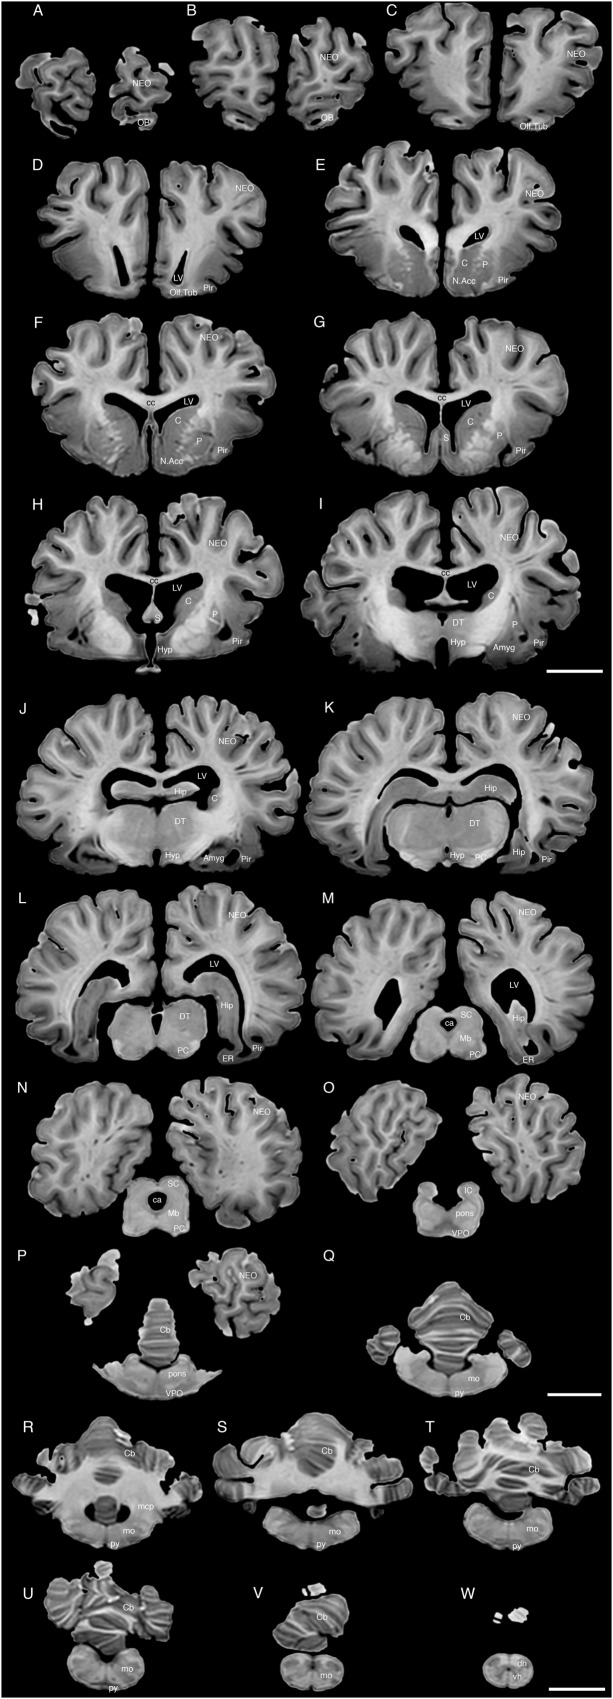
A series of coronal structural MR images through the brain of the white rhinoceros (*C. simum*). (A) is the most rostral section and (W) is the most caudal section, with each section having a thickness of 2 mm and each section being 6 mm apart. Note the typically mammalian topography of the various regions and structures of the brain. Scale bar = 2 cm. Amyg, amygdaloid body; C, caudate nucleus; ca, cerebral aqueduct; Cb, cerebellum; cc, corpus callosum; dh, dorsal horn; DT, dorsal thalamus; EC, entorhinal cortex; Hip, hippocampus; Hyp, hypothalamus; IC, inferior colliculus; LV, lateral ventricle; N.Acc, nucleus accumbens; NEO, neocortex; Mb, midbrain; mcp, middle cerebellar peduncle; mo, medulla oblongata; OB, olfactory bulb; Olf. Tub, olfactory tubercle; P, putamen; PC, cerebral peduncle; Pir, piriform cortex; py, pyramidal tract; S, septal nuclear complex; SC, superior colliculus; vh, ventral horn; VPO, ventral pontine nucleus.
